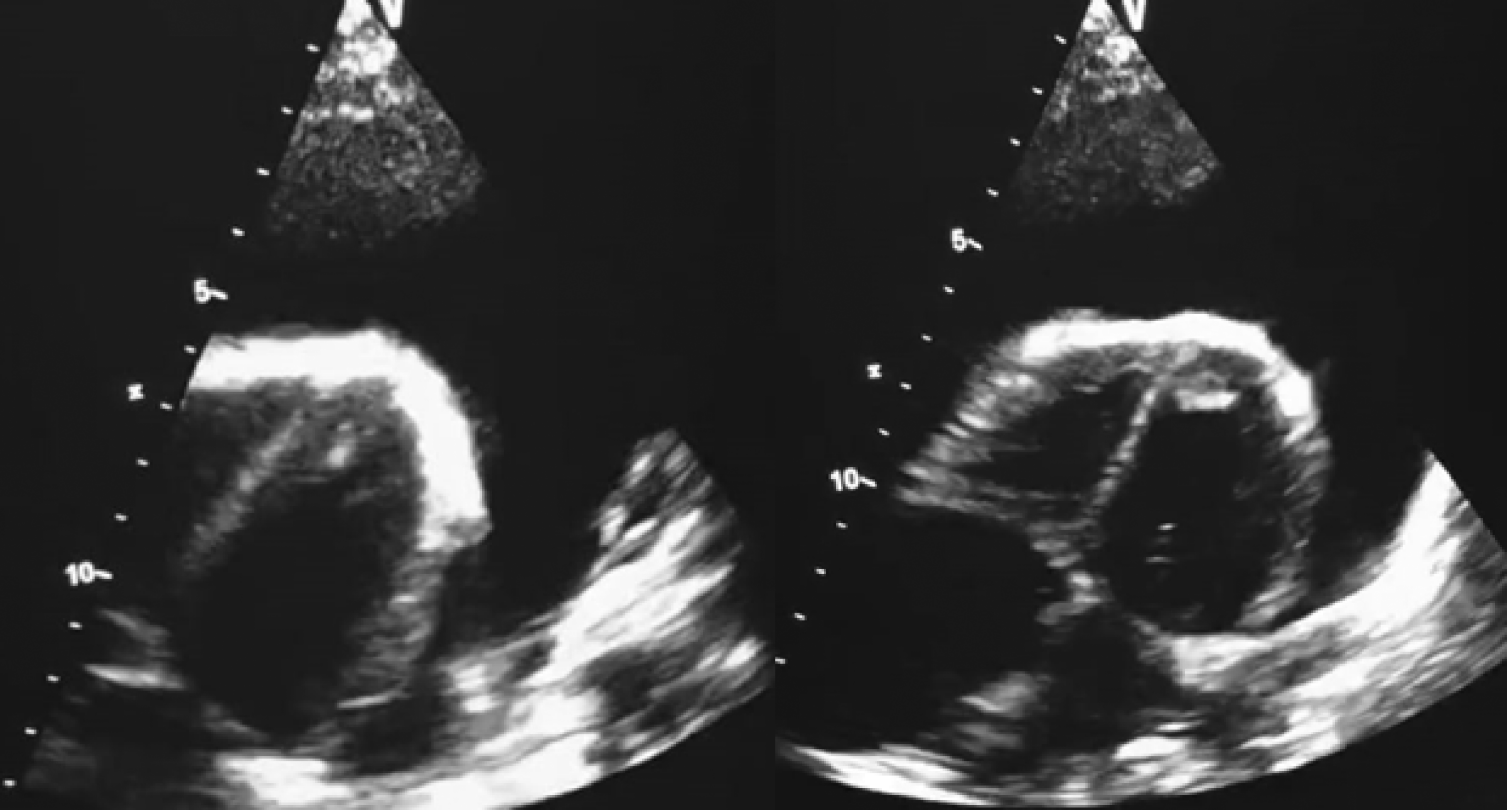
An echocardiogram demonstrates a severe circumferential pericardial effusion.
